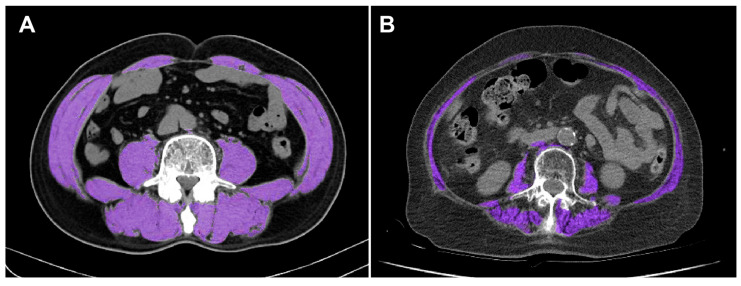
Example of CT-based evaluation of skeletal muscle at the L3 level in two representative patients: (A) non-sarcopenia and (B) sarcopenia. Skeletal muscle areas are highlighted in purple.
